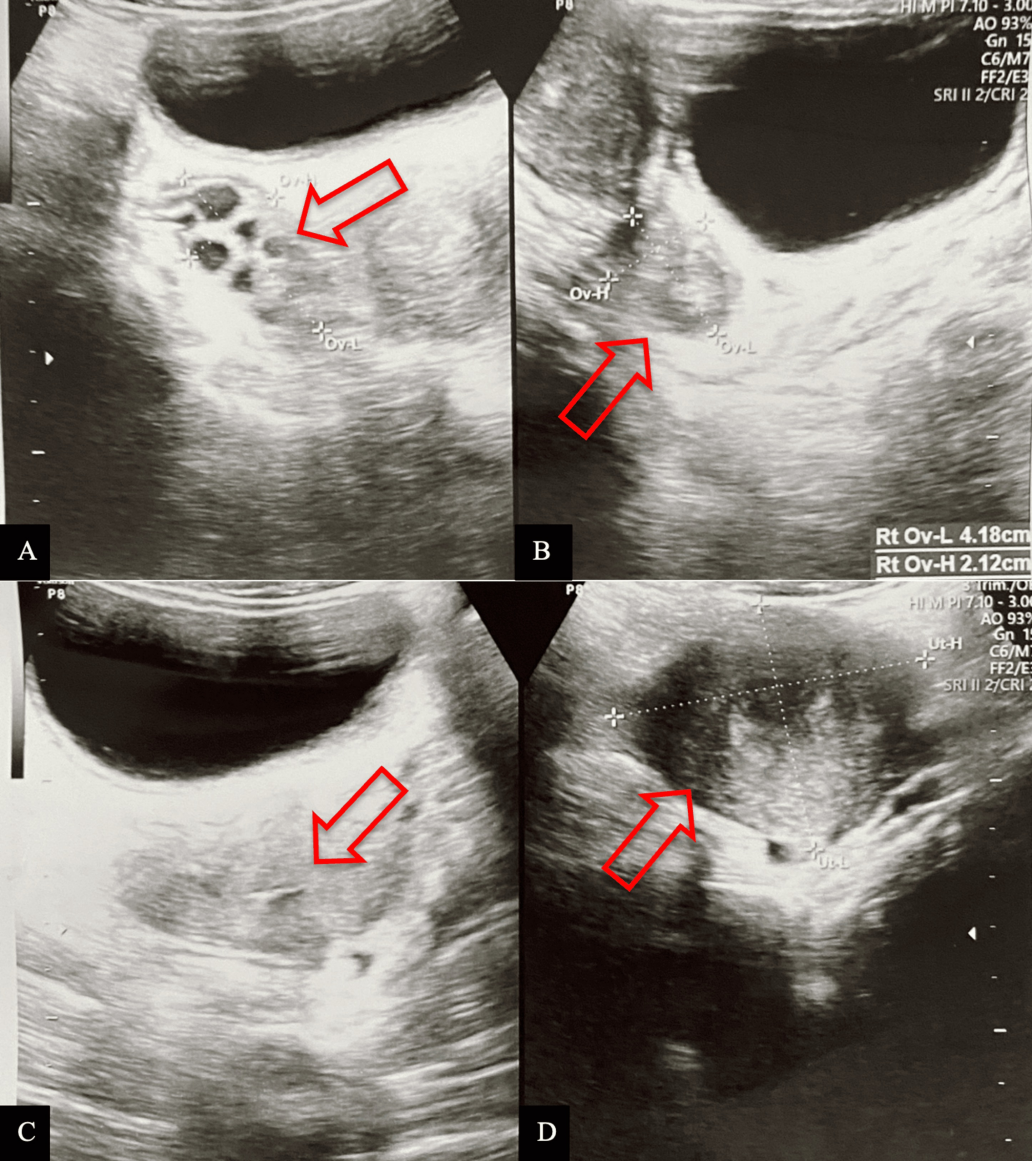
Transabdominal ultrasound shows a polycystic structure suggestive of residual blood on the left ovary (A), a normal right ovary (B), an empty canalis vaginalis (C), and an empty uterine cavity (D) shown by a red arrow.
